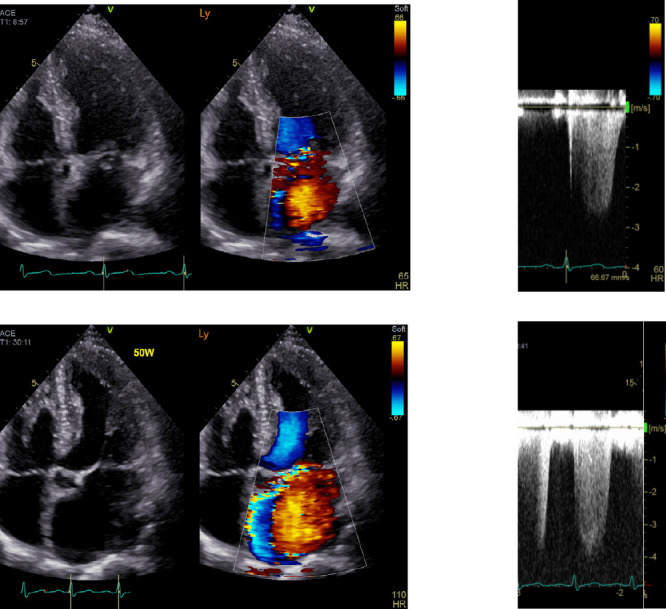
Representative case of exercise-induced PH Apical four-chamber image with color Doppler (upper left) and TR flow (upper right) at rest. Increased mitral regurgitation (lower left) and TRPG suggesting PH (lower right) during exercise. TR, tricuspid regurgitation; TRPG, tricuspid regurgitation pressure gradient; PH, pulmonary hypertension.
